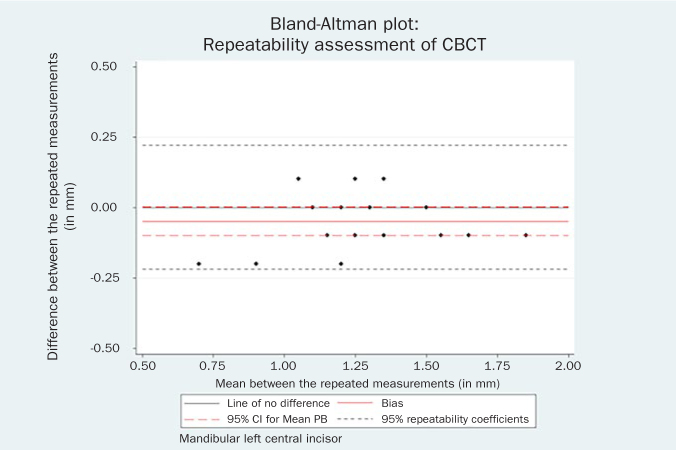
Bland-Altman plot of the repeated CBCT measurements for the mandibular left central incisor.
